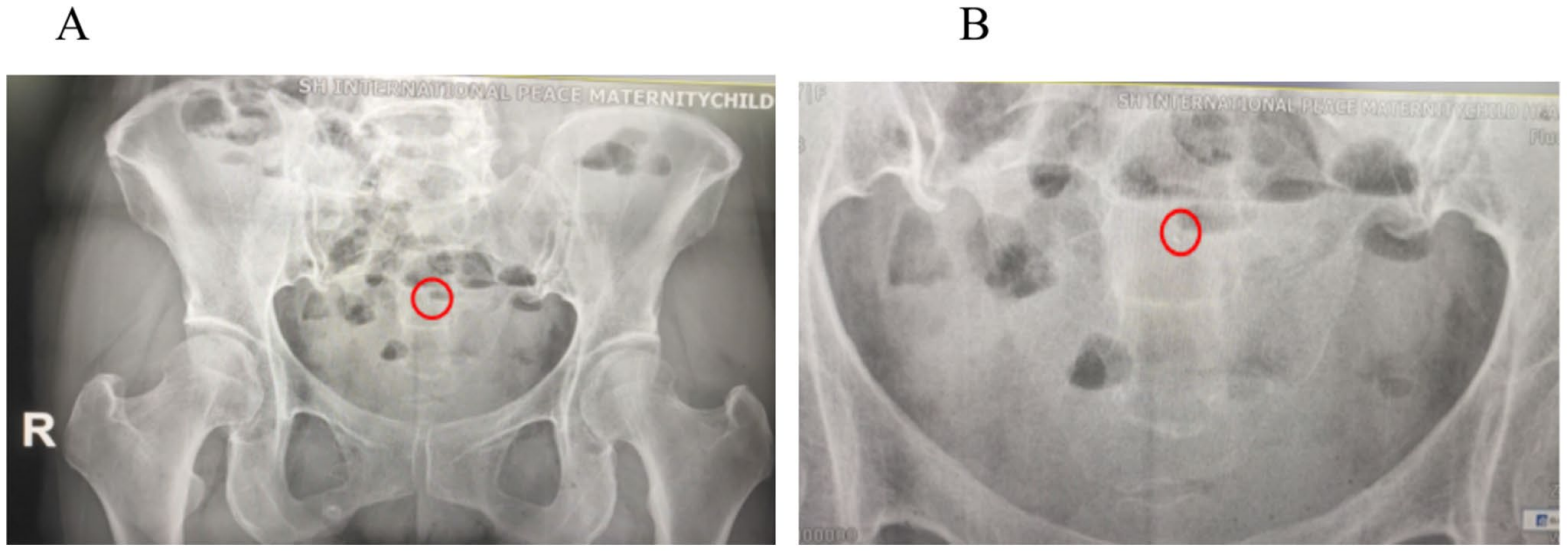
(A) Postoperative abdominal plain film: A punctate, slightly high-density shadow approximately 2 mm in length was observed 5.4 cm above the pubic symphysis in the pelvic cavity (red circle). (B) An enlarged high-density shadow of 2 mm (red circle).
